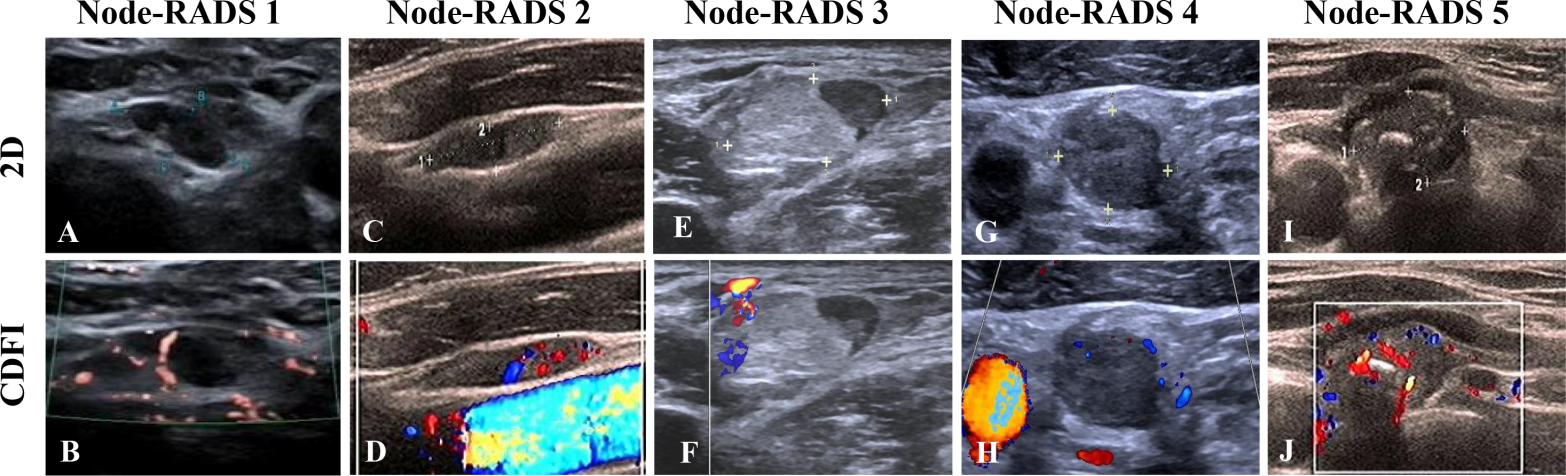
Representative cervical LN of different Node-RADS scores. (A, B) Node-RADS score 1: short axis = 4 mm (0 points), homogeneous texture (0 points), smooth borders (0 points), and kidney-bean-like shape with fatty hilum (0 points); (C, D) Node-RADS score 2: short axis = 5 mm (0 points), heterogeneous texture (1 point), smooth border (0 points), and kidney-bean-like shape without fatty hilum (0 points); (E, F) Node-RADS score 3: short axis = 10 mm (1 point), heterogeneous texture (1 point), smooth border (0 points), and oval shape without fatty hilum (0 points); (G, H) Node-RADS score 4: short axis = 9 mm (0 points), heterogeneous (1 point), irregular border (1 point), and spherical without fatty hilum (1 point); (I, J) Node-RADS score 5: short axis = 16 mm (1 point), calcification (3 points), irregular border (1 point), and spherical without fatty hilum (1 point). LN, lymph node; CDFI, Color Doppler flow imaging; Node-RADS, Node Reporting and Data System.
